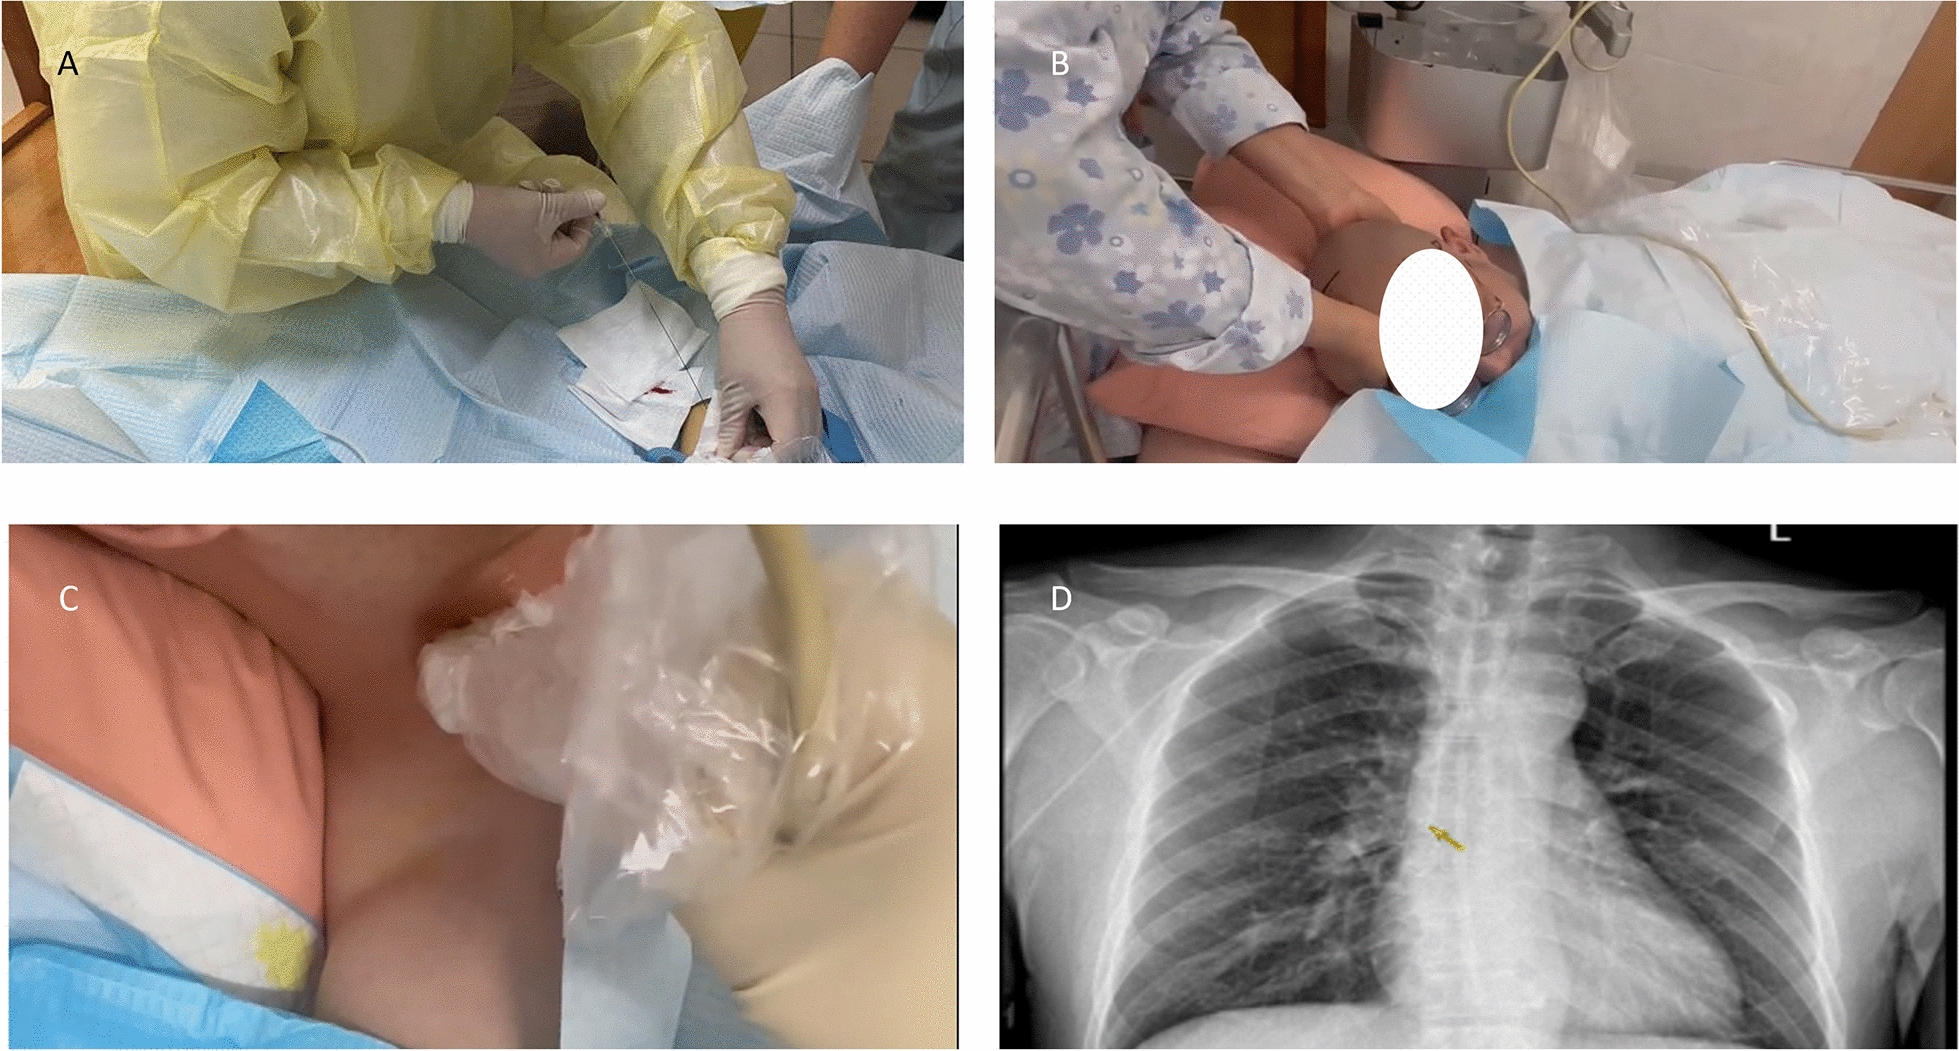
A Feeding guide wire. B Assistant helping the patient keep his chin close to his chest during delivery. C B-ultrasound examination of the blood vessels in the neck after insertion. D Infusion therapy after chest X-ray confirmation
